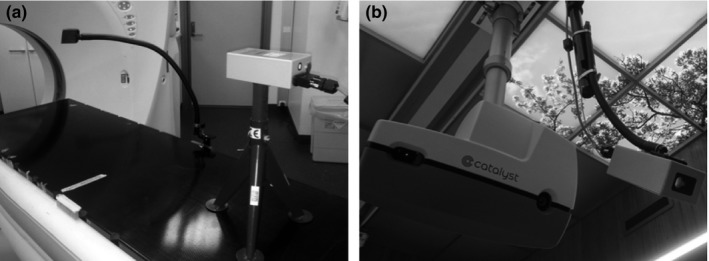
(a) Laser measurer pointing at Flexarm mirror during acquisition of planning CT. (b) Catalyst and laser measurer mounted on the ceiling in the treatment room.
